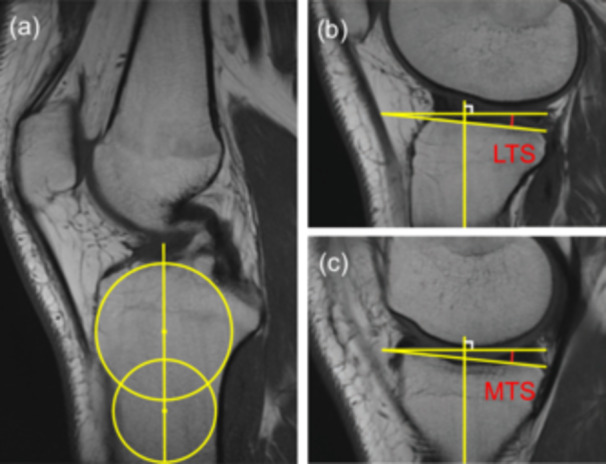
Magnetic resonance images demonstrating the measurement of tibial slopes. (a) The proximal tibial anatomic axis was determined on the central sagittal slice by connecting the centres of two circles placed at the tibial metaphysis. On midsagittal slices of the lateral (b) and medial (c) compartment, lateral tibial slope (LTS) and medial tibial slope (MTS) were measured as complementary angles (red arcs) between the tangential lines of the tibial plateau and the proximal tibial anatomic axis.
